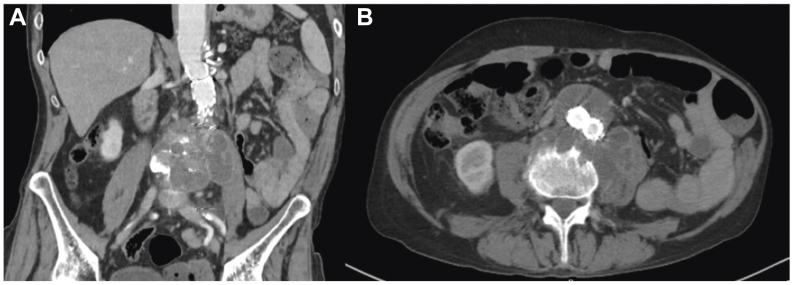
(A and B) Computed tomography angiography (CTA) revealing a 7.5 × 6.0 cm loculated periaortic fluid collection eroding L3-L4 vertebrae and extending down the left psoas muscle.
